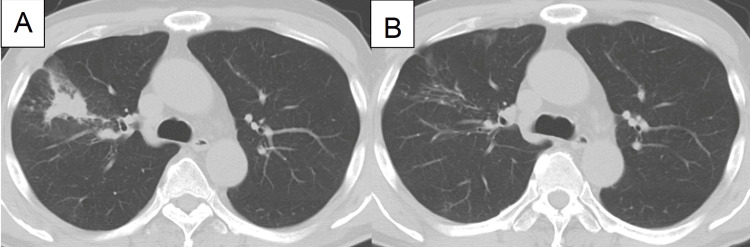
Radiological findings in Case 2. Chest CT showed consolidation in  the anterior segment of the right upper lobe (S3) (D) and the opacity was improved spontaneously (E).
